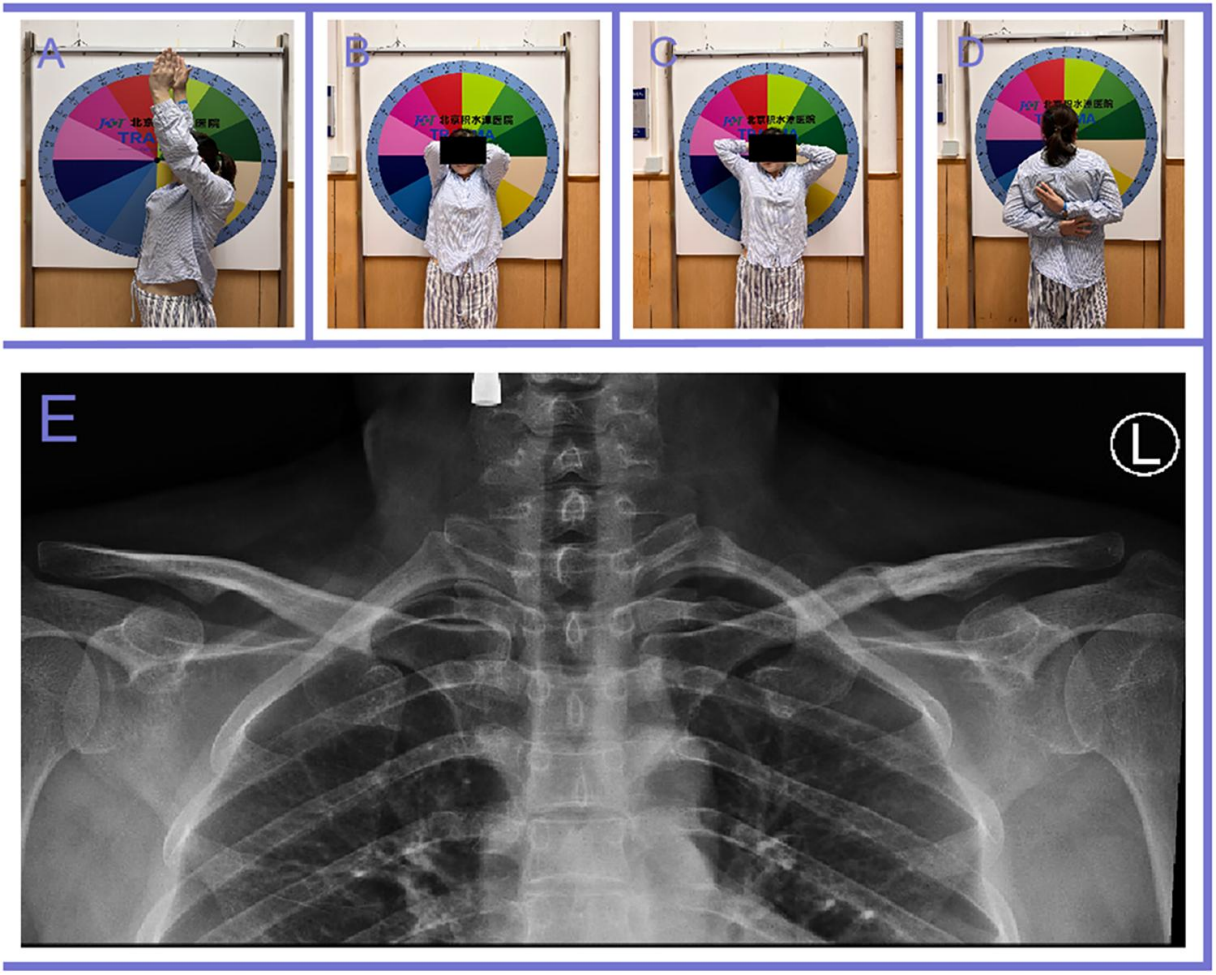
Good range of movement of the shoulders at the time of nail removal (A)–(D). x-ray imaging after nail removal revealed notable bone remodeling with reduced callus formation at the fracture site compared to the findings observed at the onset of the brachial plexus injury (E).
